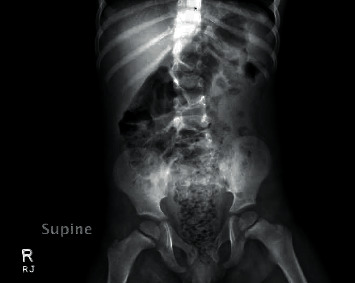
Abdominal X-ray, supine view. There is a nonobstructive gas pattern with moderate stool burden, primarily in the rectosigmoid colon and to a lesser extent in the ascending colon.
